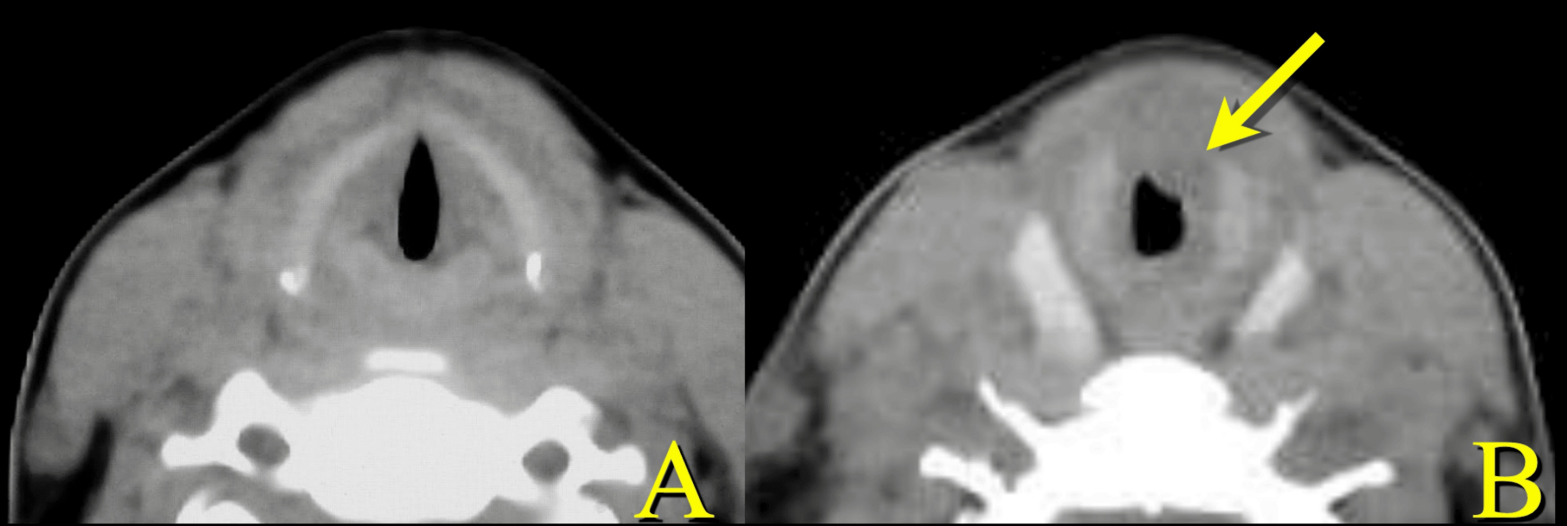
Axial CT at the initial presentation. The glottis appears intact (A). A slightly caudal section (B). Thickening of the anterior tracheal wall (arrow) suggests subglottic stenosis (B).
